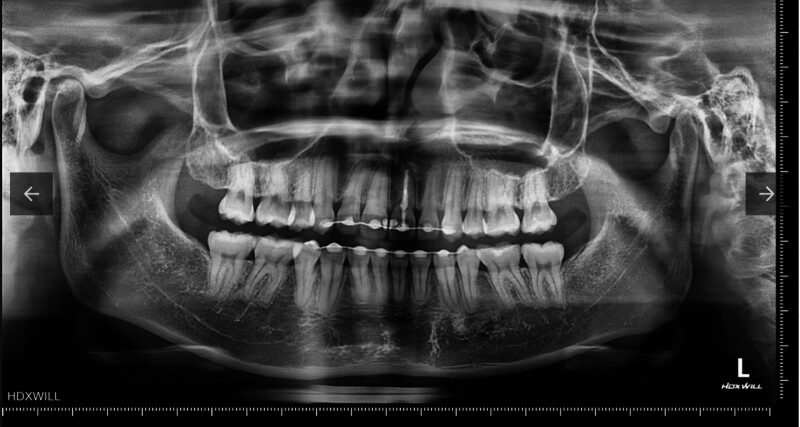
Preoperative radiograph. The left central incisor had been endodontically treated, showing no signs or symptoms of infection.
